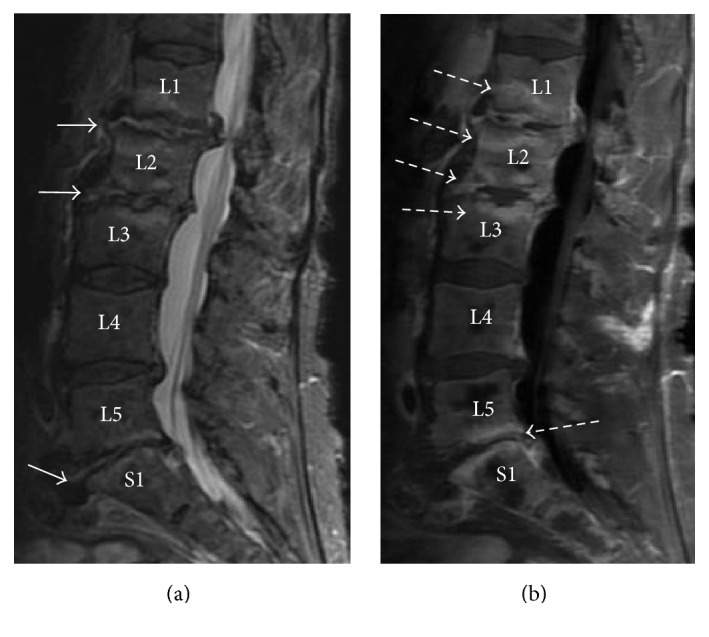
Prevertebral osteomyelitis, discitis, and soft-tissue abscess seen on sagittal MRI imaging with and without contrast*. (a) Sagittal T2 STIR showing high signal consistent with fluid in the L1-2, L2-3, and L5-S1 disc spaces with endplate edema (white arrows). (b) Sagittal T1 fat saturation obtained after contrast shows extensive enhancement centered around the L1–3 and L5-S1 disc spaces consistent with discitis and osteomyelitis (dashed arrows).
